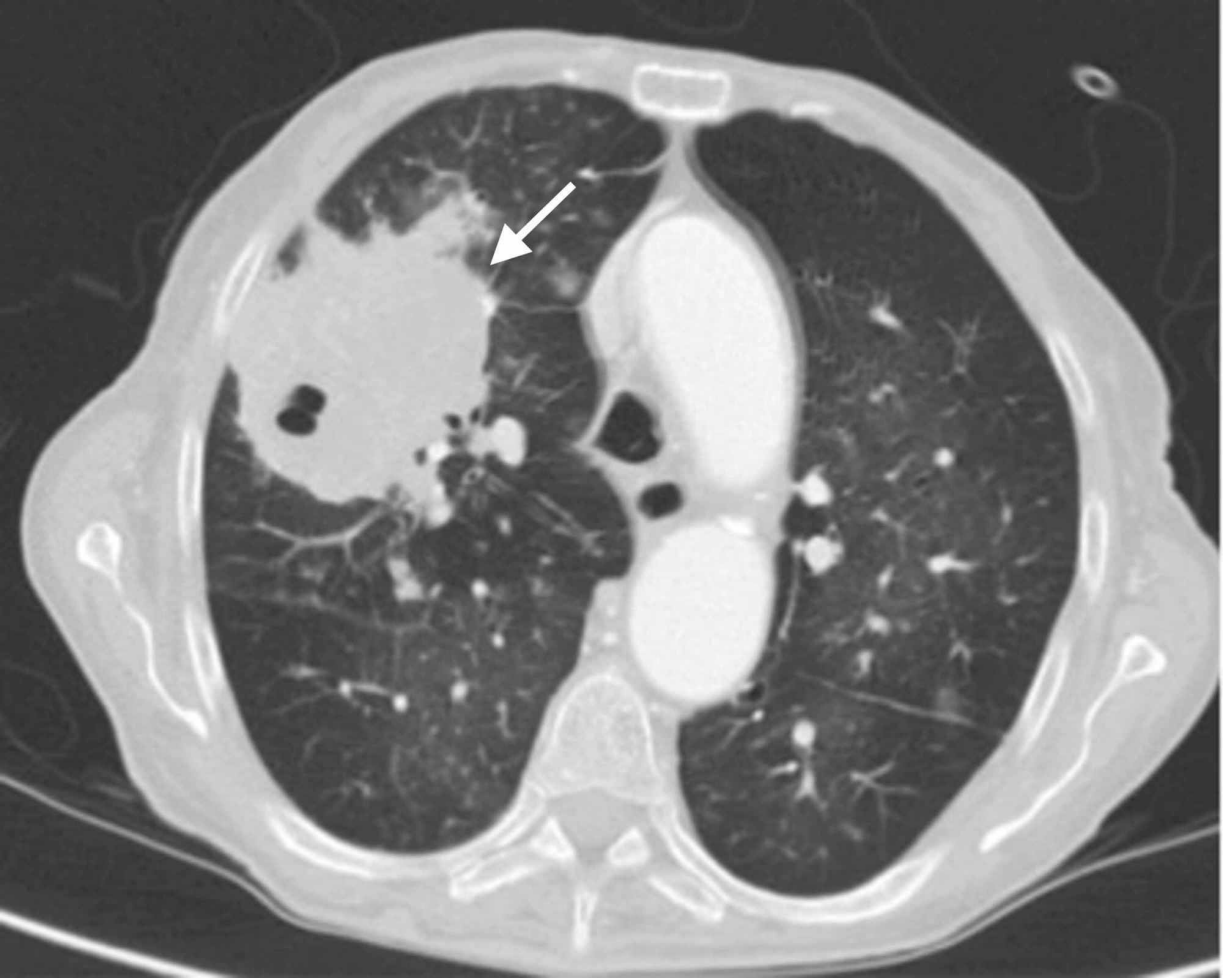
CT scan Areas of consolidation in the right upper lobe with gas-fluid levels inside, suggesting necrotizing pneumonia (arrow).
